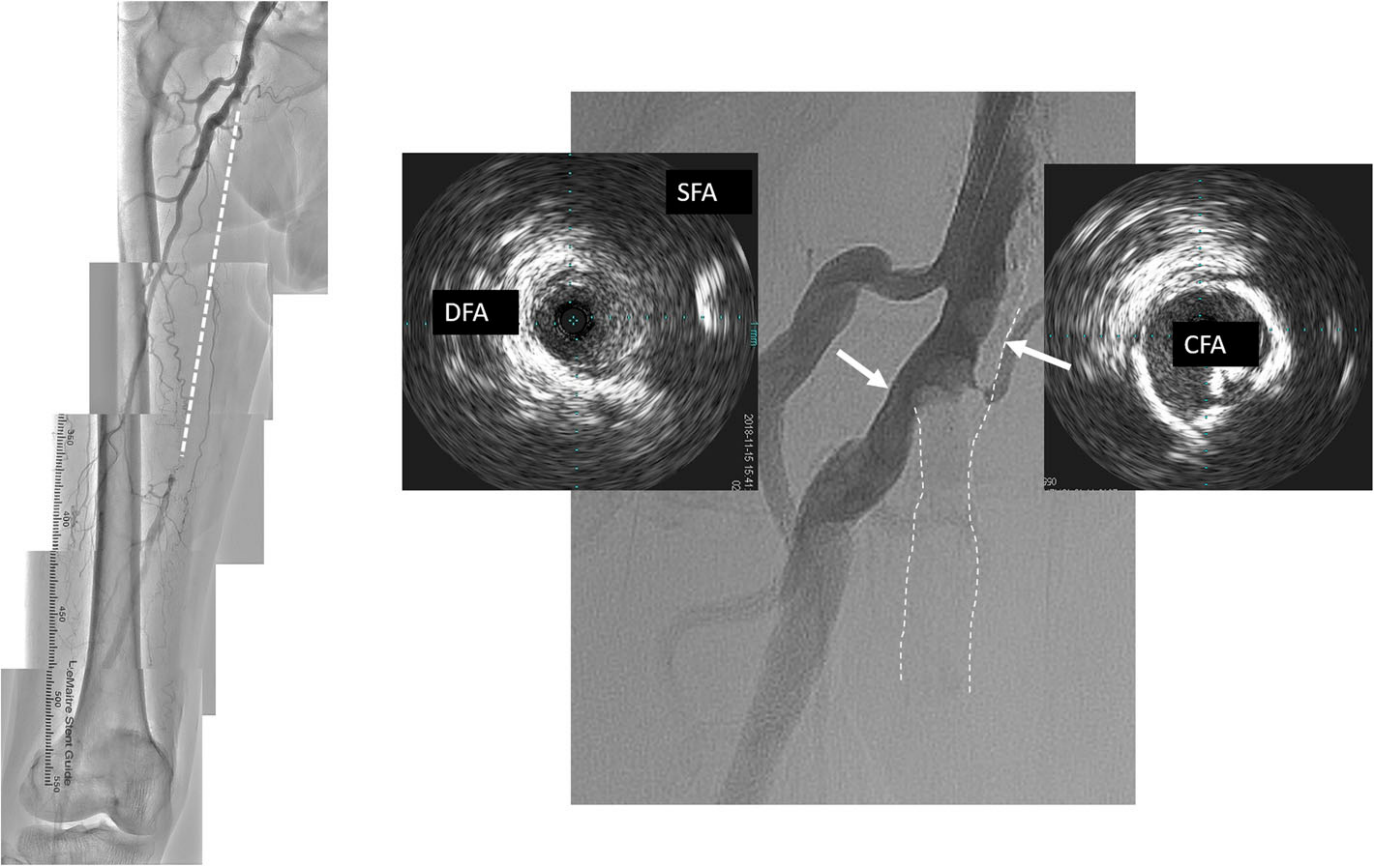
Angiography revealed a total occlusion in the right SFA. IVUS from the DFA revealed that the calcified plaque at the ostium of the SFA protruded into the CFA. SFA, superficial femoral artery; IVUS, Intravascular ultrasound; DFA, deep femoral artery; CFA, common femoral artery
